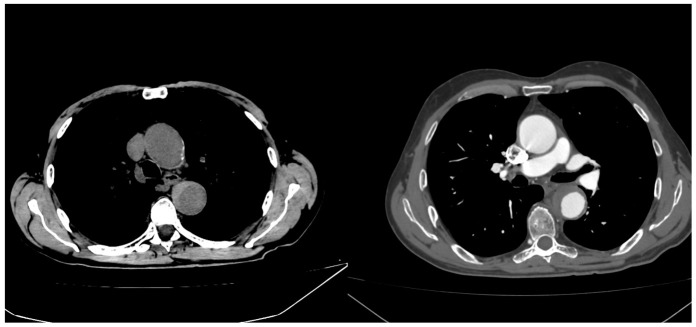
Emergency CT angiography ((left): non-contrast phase; (right): arterial phase) demonstrates a fusiform aneurysm of the descending thoracic aorta, with intraluminal hyperdensity on non-contrast images and active contrast extravasation on arterial-phase imaging. These findings are consistent with acute hemorrhage and aneurysmal instability, underscoring the pivotal role of CTA in detecting life-threatening complications.
